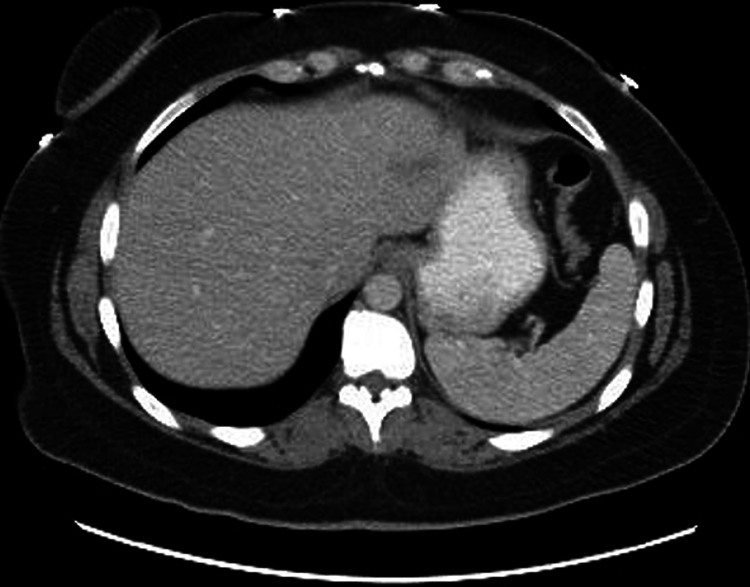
CT abdomen with resolving hepatic abscess in the left lower lobe
